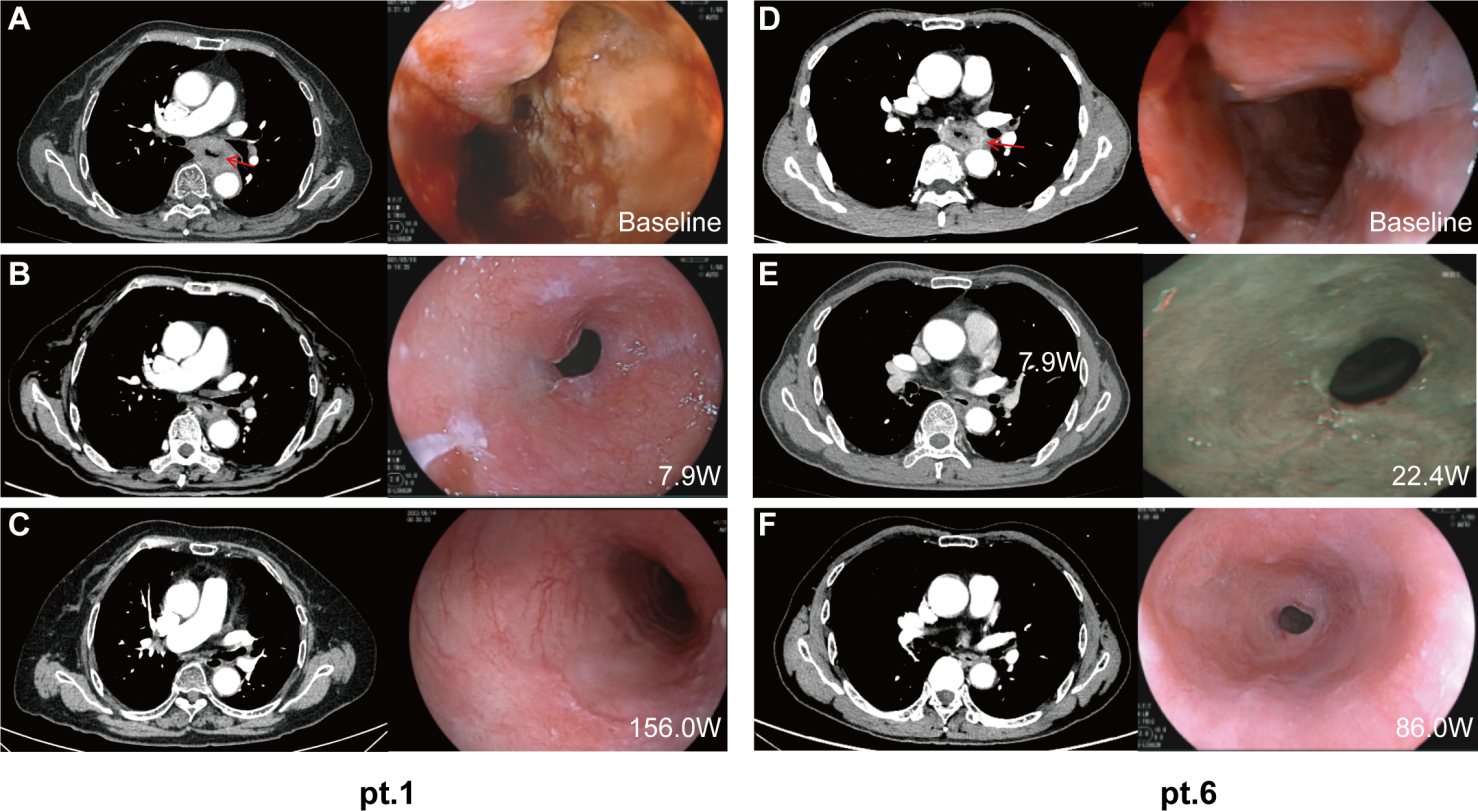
Radiology and endoscopic images of the primary tumor during treatment. Representative computed tomography and endoscopy images of patient 1 (quick responder) and patient 6 (delayed responder) at baseline (A, D), at the time of clinical complete response (B, E), and at the most recent follow-up (C, F). *W*, weeks from the first dose.
